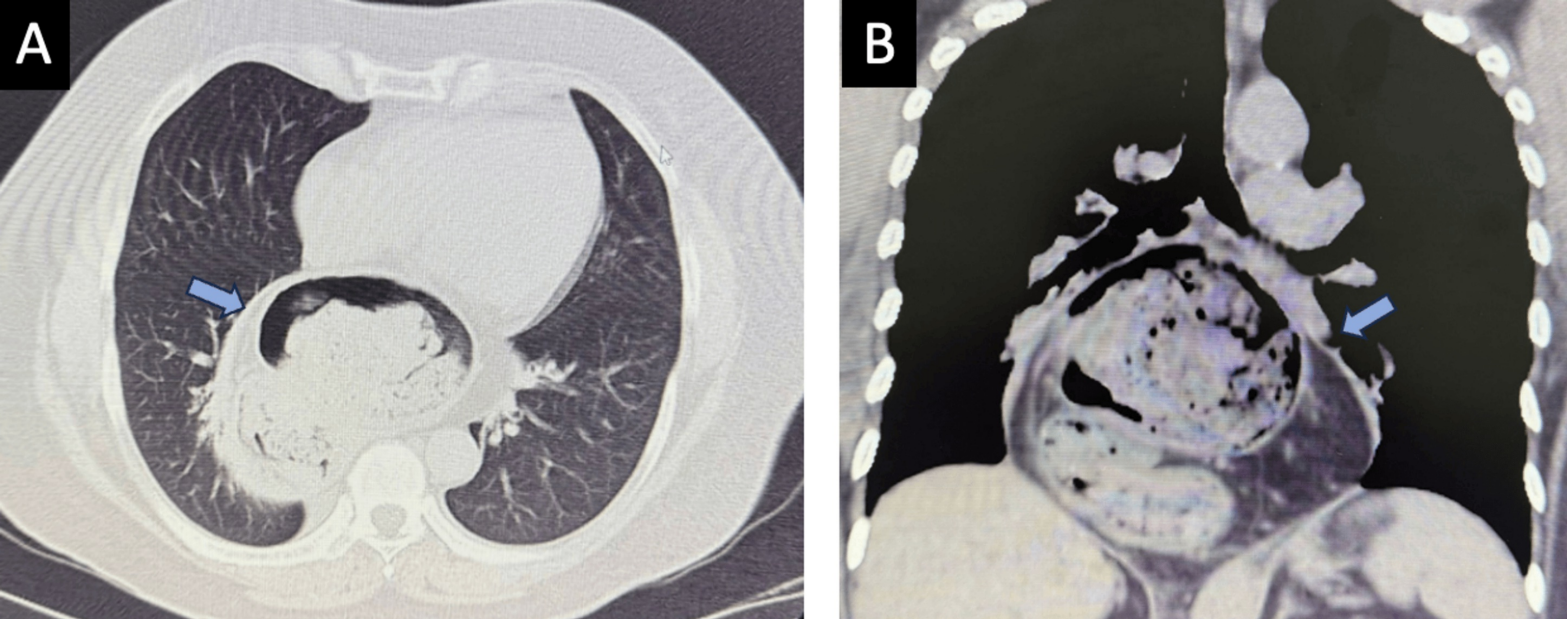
Thoracoabdominal computed tomography (A-B) Type IV hiatal hernia involves the gastric fundus, a portion of the splenic flexure of the colon, and the upper pole of the spleen.
